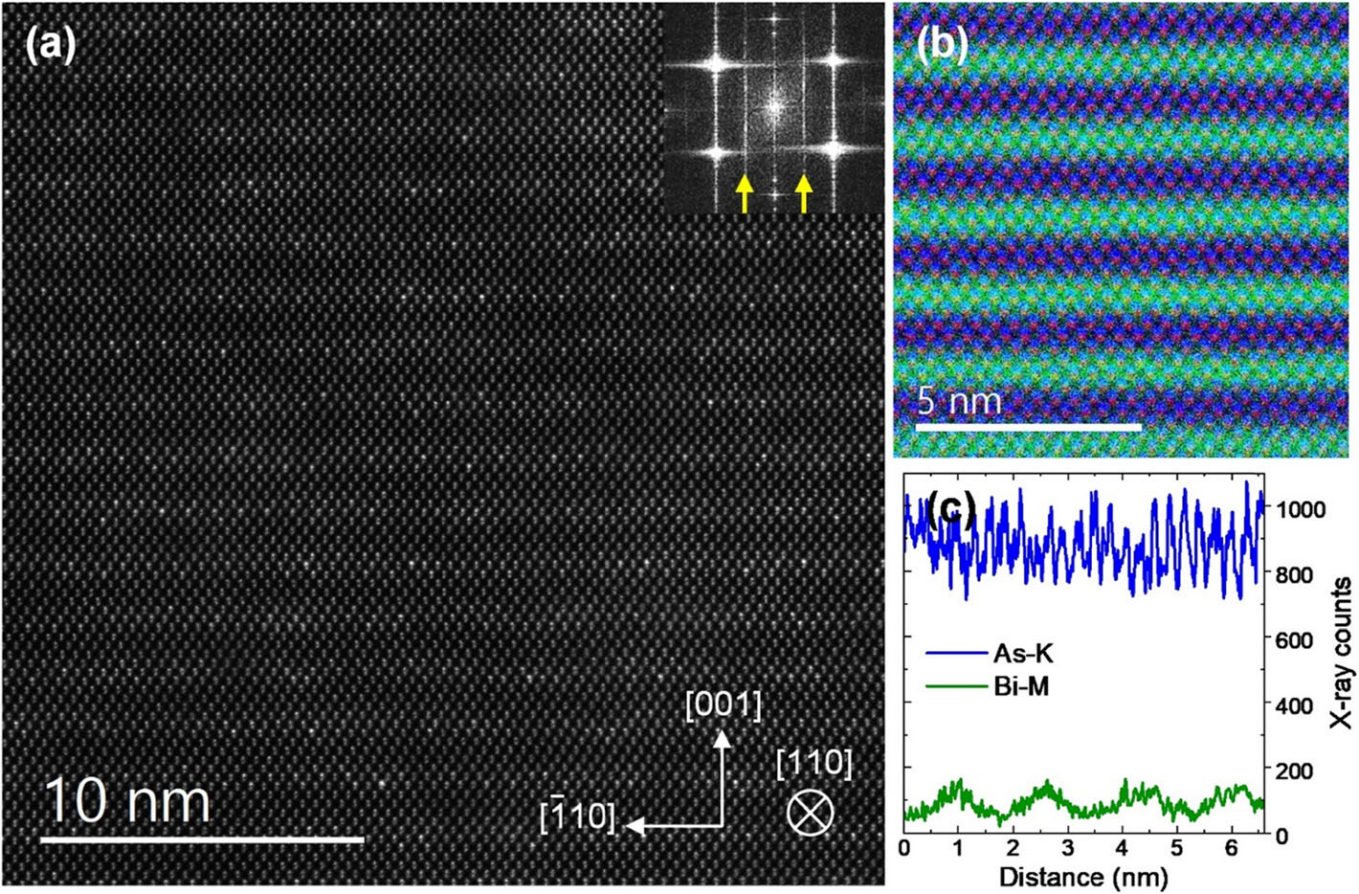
a HAADF image of the VCM GaAsBi sample S3. Bi concentration modulations along the growth [001] direction are visible, as well as CuPt_B_ ordering within the Bi-enriched planes. Inset shows Fourier transform with reminiscent CuPt_B_ ordering modulated by the VCM. b A combined Wiener filtered elemental EDX image of the sample with normalized X-ray counts for each element, Bi-green, As-Blue, and Ga-red. c Vertical X-ray count profile extracted from 3 × 3 binned raw As-K and Bi-M signals, horizontally summed within a 5 nm window
